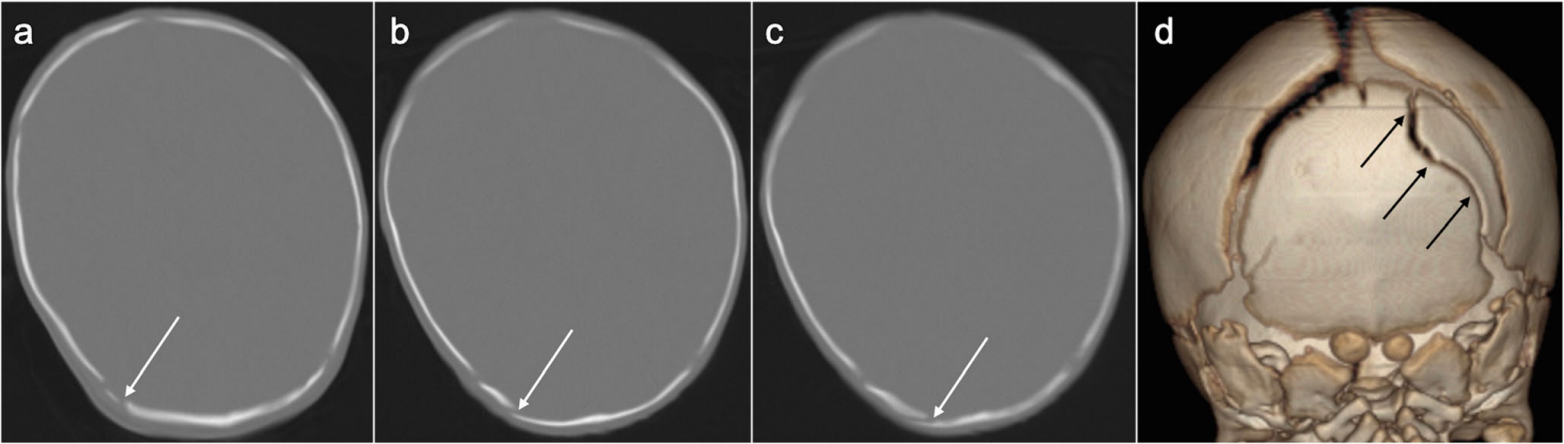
a–c Selected axial slices (inferior to superior) on bone windows from the head CT examination in the same infant as Fig. 3 which demonstrate a cortical irregularity involving the right occipital bone (white arrows), suggestive of a fracture. However, there is no associated soft tissue swelling and on the 3D reconstruction (d); the cortical irregularity is curvilinear in appearance rather than linear, the latter being more typical of a fracture. The location, adjacent to the lambdoid suture, its appearance, and the absence of soft tissue swelling suggest this is more in keeping with an accessory lambdoid suture (black arrows)
